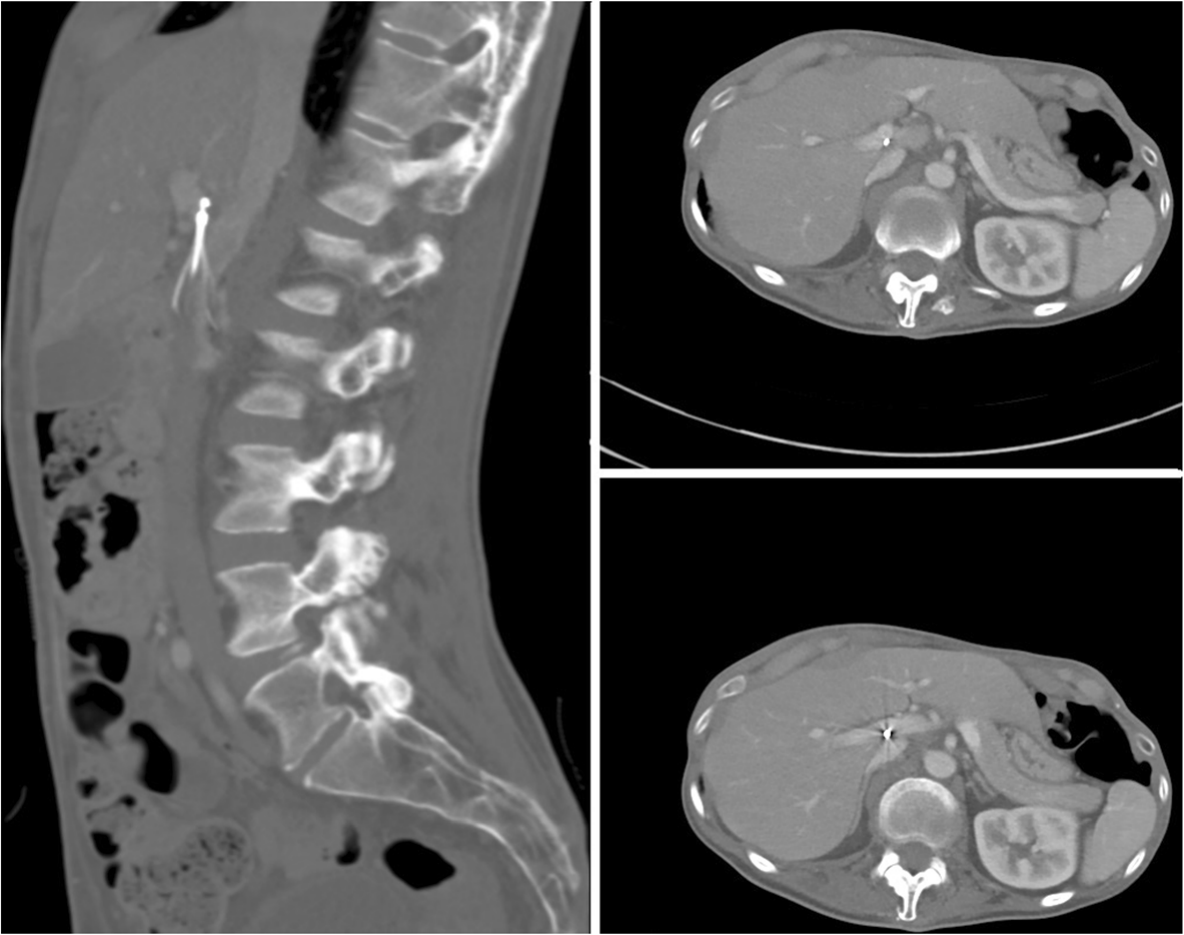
This patient had a suprarenal Günther Tulip IVC filter (Cook, Bloomington, IN) placed at OSH approximately 5 years prior to obtaining this CT. Images showed the filter hook outside the lumen of the IVC and within hepatic parenchemya and possibly into the portal vein. Given that the patient was asymptomatic and that filter removal could lead to signfiicant complications it was elected not to pursue retrieval
